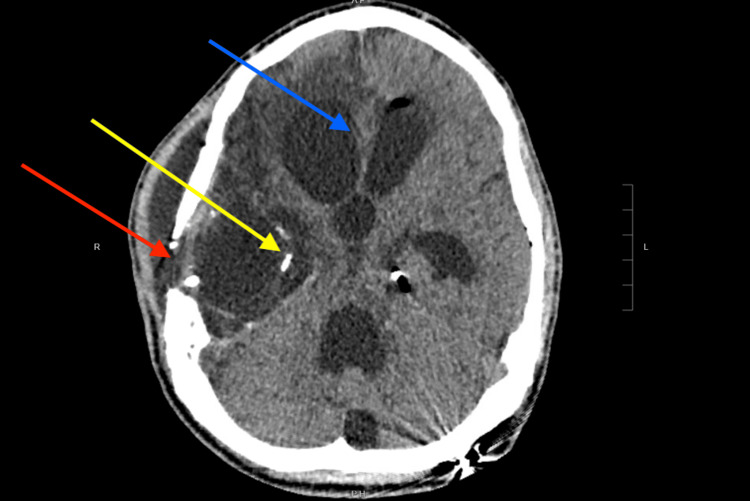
Initial non-contrast head CT Red arrow: open temporal bone defect. Yellow arrow: metallic bullet fragments. Blue arrow: subfalcine herniation.
